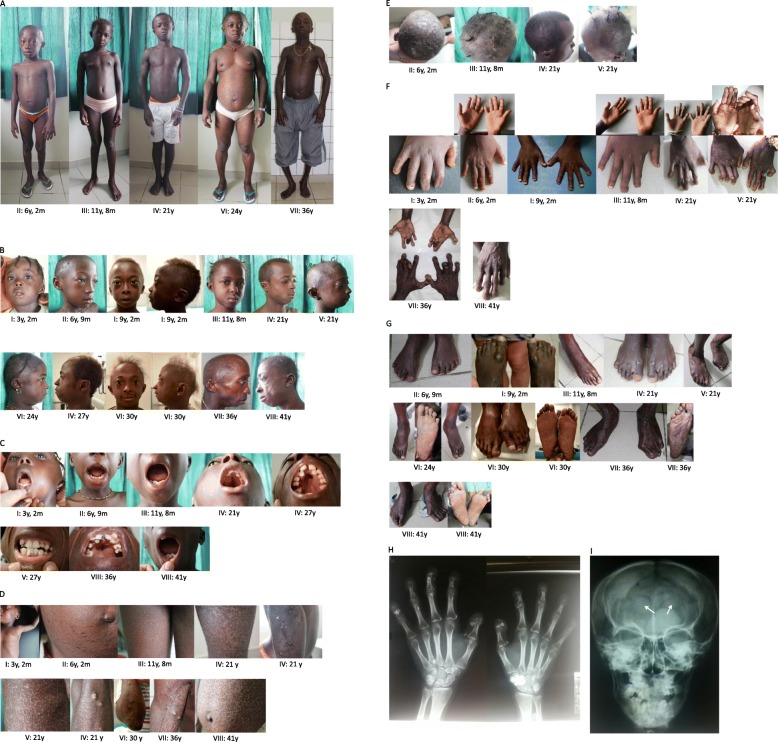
Images of individuals with MADB at chronological ages. Photographic images of a total body showing typical lipodystrophy; b facial features showing facial lipoatrophy, and mandibular hypoplasia; c oral region and teeth showing dental crowding, high palate; d skin showing calcified skin nodules and mottled, sclerodermic and atrophic skin in from left to right: patient, abdomen, legs (patient III, IV), arms (patients IV, V, IV, VI, VII) and abdomen e skull showing brittle and/or sparse hair; f hands and g feet showing acro-osteolysis of distal phalanges, shortened phalanges, joint contractures, hypoplastic nails, palmar and plantar atrophy of the hand and feet and calluses. X-rays of patient III showing h acro-osteolysis of the distal phalanges and i diminished occipital ossification (indicated by white arrows)
